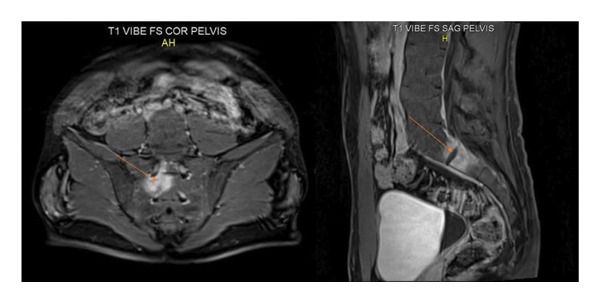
Dynamic MRI showing edema centered on the S1‐S2 disc, lateralized to the right of the midline, extending into the right S1 foramen.
